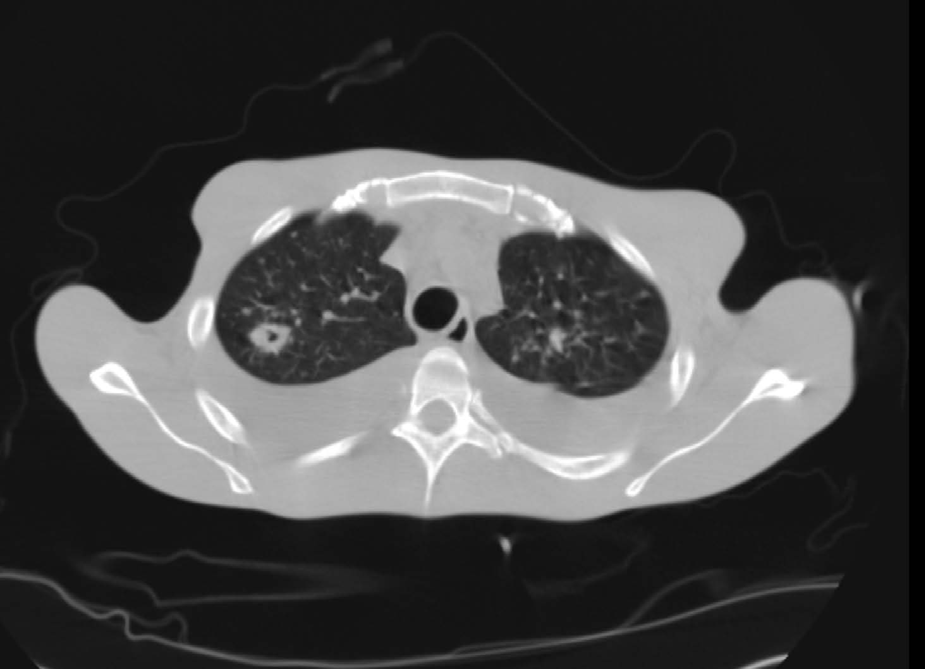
Computed tomography of the chest with bilateral pleural effusion and multiple nodular lesions, one of which is excavated.
